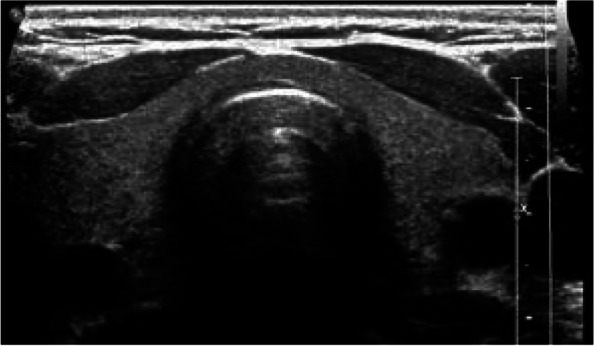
Gray-scale ultrasound images show homogeneous thyroid parenchyma in a Group III participant
